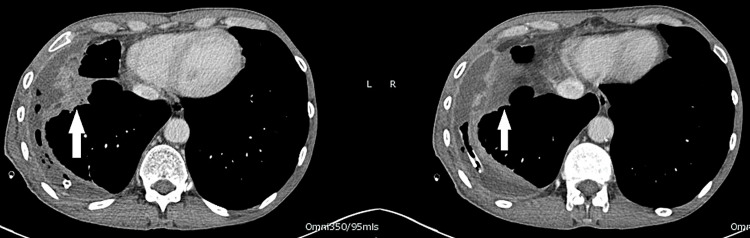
CT Thorax images showing moderate right pleural collection containing fluid and locules of gas (arrow) CT: Computed tomography
